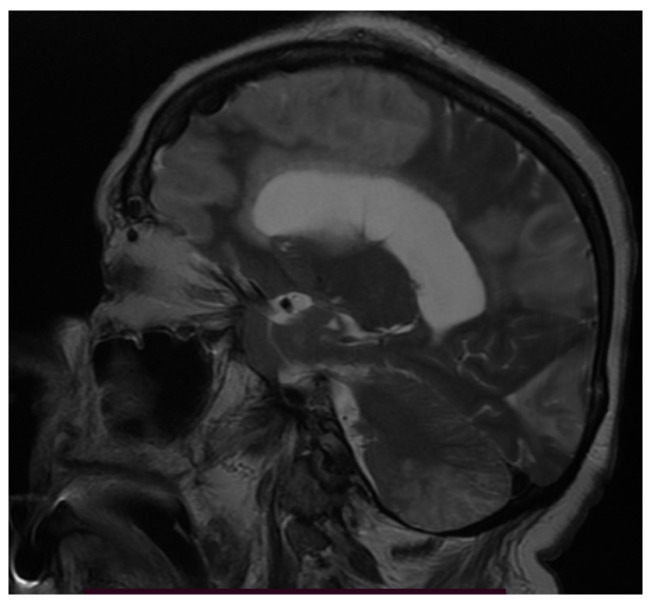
MRI sagittal T2-weighted sequence showing diffuse confluent hyperintensities in the peri-corpus callosum.
